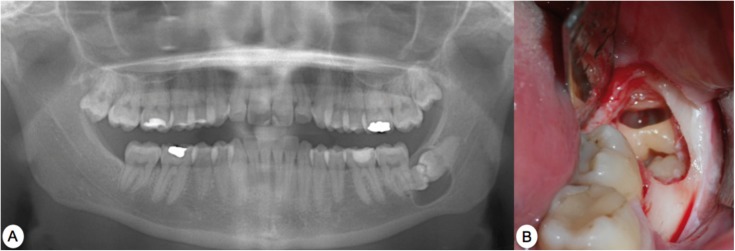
Case 2. A) Preoperative orthopantomogram. B) Vestibulo-lingual tooth section of the supernumerary molar crown.
